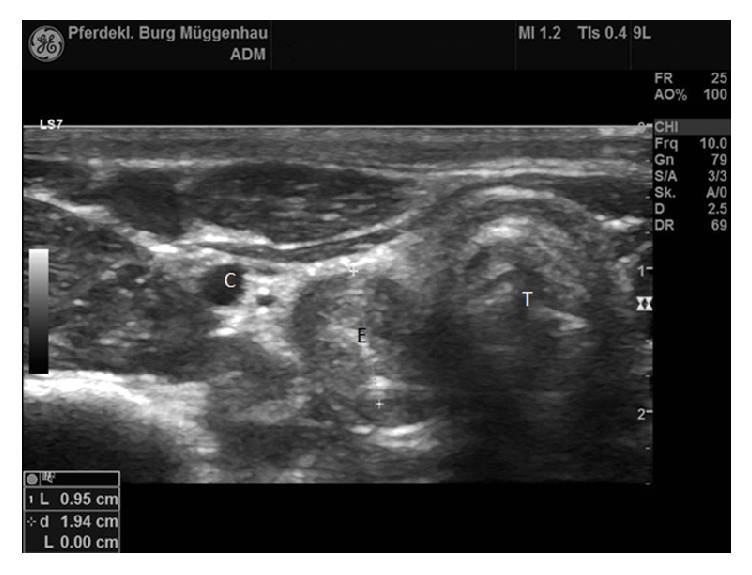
Ultrasound image of the esophagus cranial to the esophageal stricture. Esophageal diameter is 9,5 mm. Left in the image is dorsal. C: carotid artery. E: esophagus. T: trachea (probe frequency 10 MHz, depth 2,5 cm).
